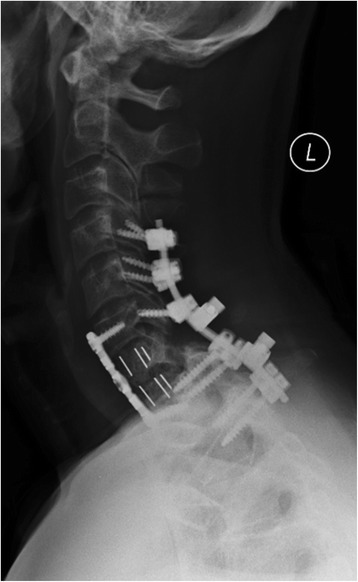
Lateral radiograph of the cervical spine after posterior C4-T1 fusion and anterior C7 corpectomy and fusion
